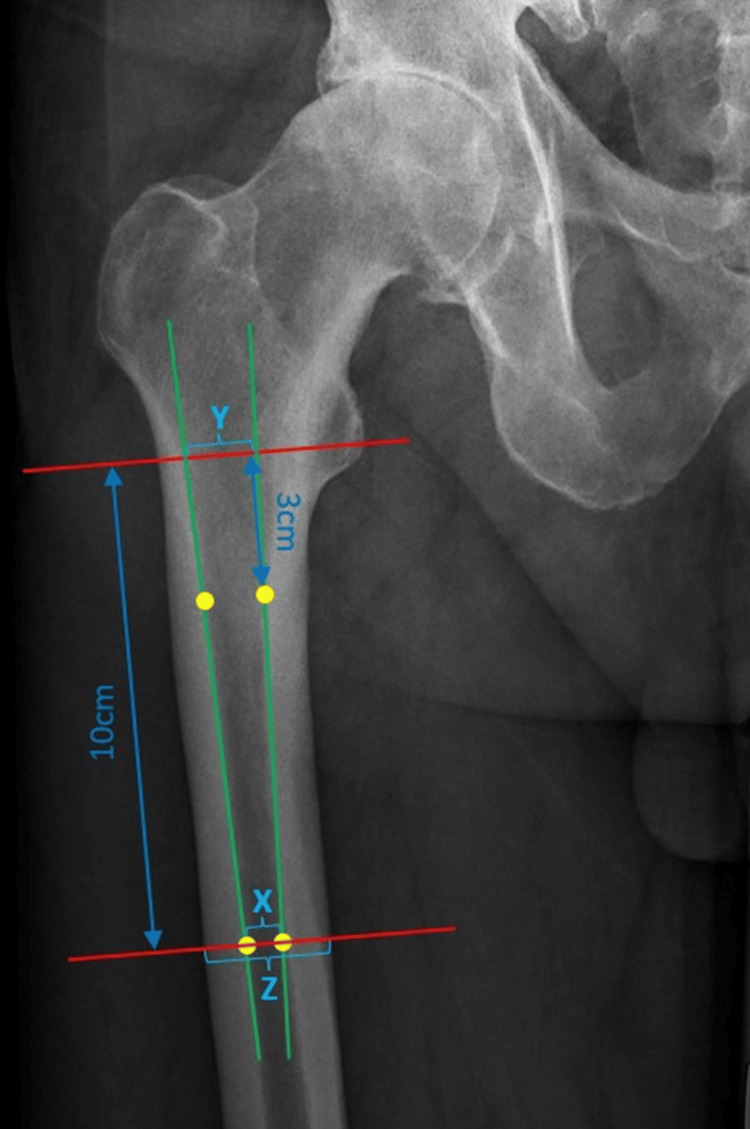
Representation of the radiological measurements taken in the study. All the measurements were made according to the method previously described by Dorr et al. [10]. In the preoperative AP radiograph of the affected hip, we identified three lines perpendicular to the long axis of the femur: the reference line crossing the apex of the lesser trochanter, the line 3 cm below the reference line, and the line 10 cm below the reference line. On both the line 3 cm and the line 10 cm below the reference line, we identified two points along the endosteal margin of the femur. By connecting the lateral and the medial points of the endosteal margin we identified the lateral and the medial endosteal lines. Next, we identified three distances: the distance (X) between two points of the endosteal margin of the femur on the line 10 cm below the reference line, the distance (Y) between the points where the lateral and the medial endosteal lines cross the reference line and the distance (Z) between two periosteal surfaces on the line 10 cm below the reference line. CC ratio was calculated by dividing the distance (X) by the distance (Y). CI was calculated by dividing the difference between the distance (Z) and the distance (X) by the distance (Z). CI, cortical index; CC, canal-to-calcar isthmus
